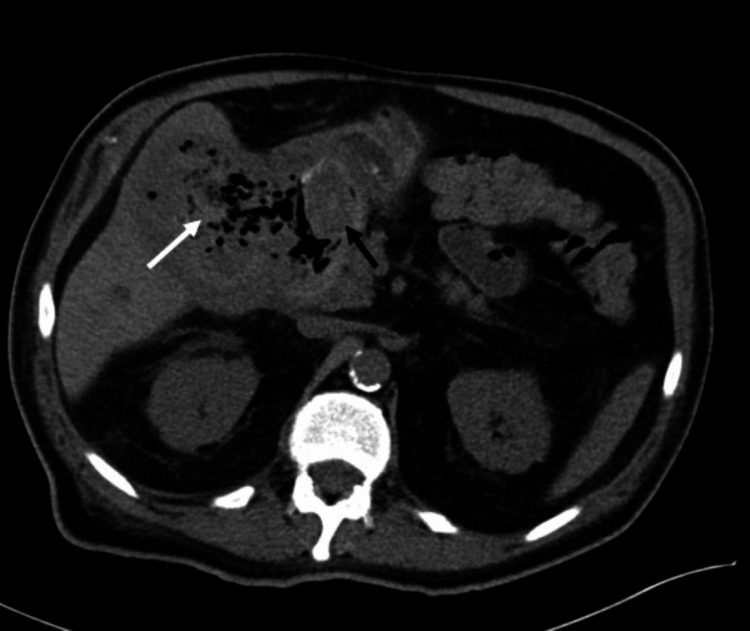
Axial CT non-contrast demonstrates gallstone (black arrow) fistulated into duodenum with gas in the gallbladder (white arrow).
